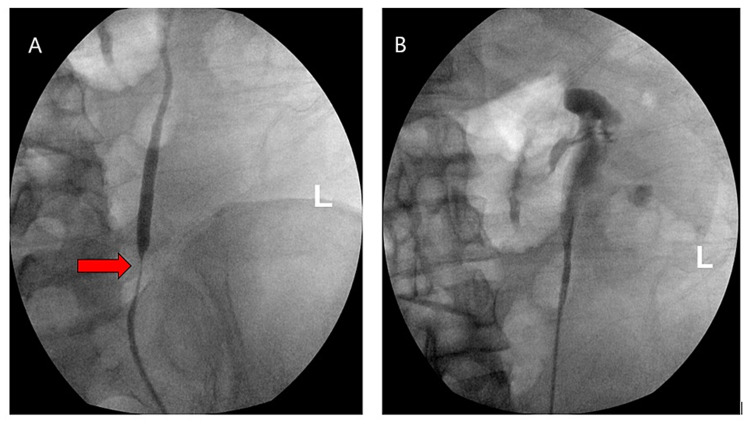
X-ray urography with left retrograde pyelogram before (A) and after stent placement (B). The red arrow in A indicates the stricture of the left ureter noted at the distal third region.
